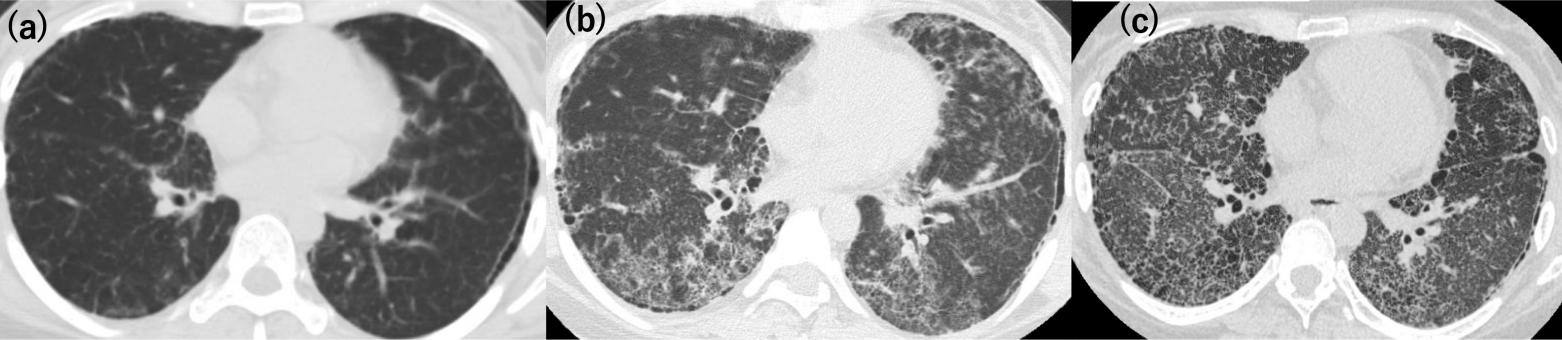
CT images of the lung. (a) age 26: Subpleural reticulation and interlobular septal thickening are noted. (b) age 36, (c) age 41. Disease progression and development of ground‐glass and nodular opacities are noted.
